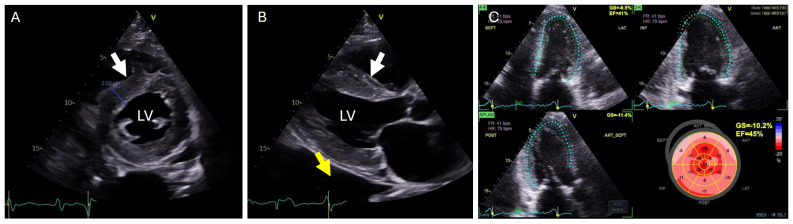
Typical echocardiographic findings in amyloidosis. (A) Parasternal short-axis view. (B) Parasternal long-axis view. (C) Three-dimensional apical long-axis view, along with strain measurement with reduced longitudinal strain and typical bullseye plot with “apical sparring”. White arrow: septal thickening up to 2 cm. Yellow arrow: pleural effusion. LV: left ventricle.
